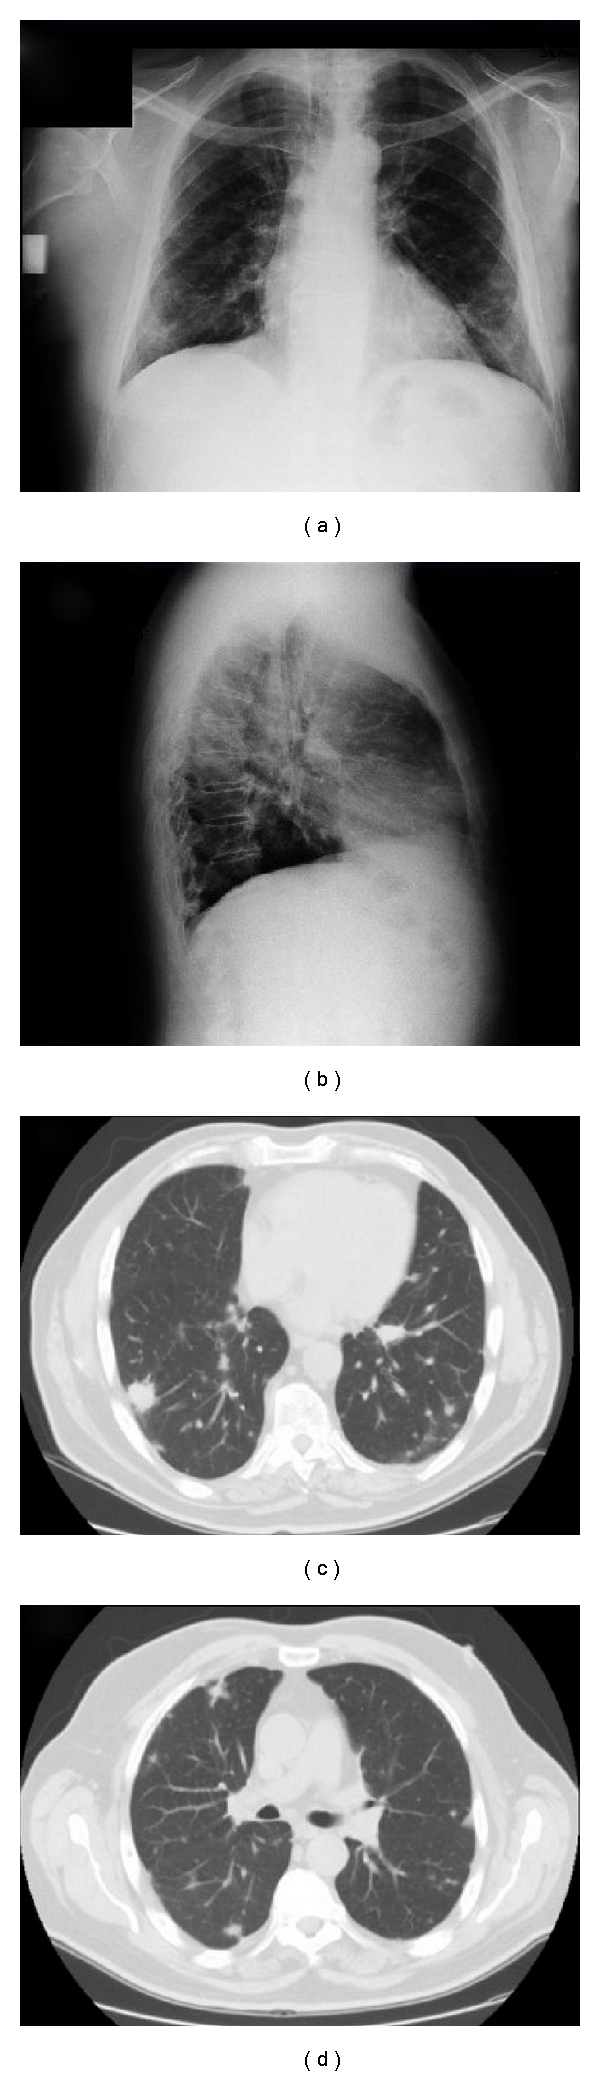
The chest radiography (a, b) and a computed tomography of the chest (performed with iodine intravenous contrast medium) (c, d) showed the presence of multiple bilateral pulmonary nodules.
